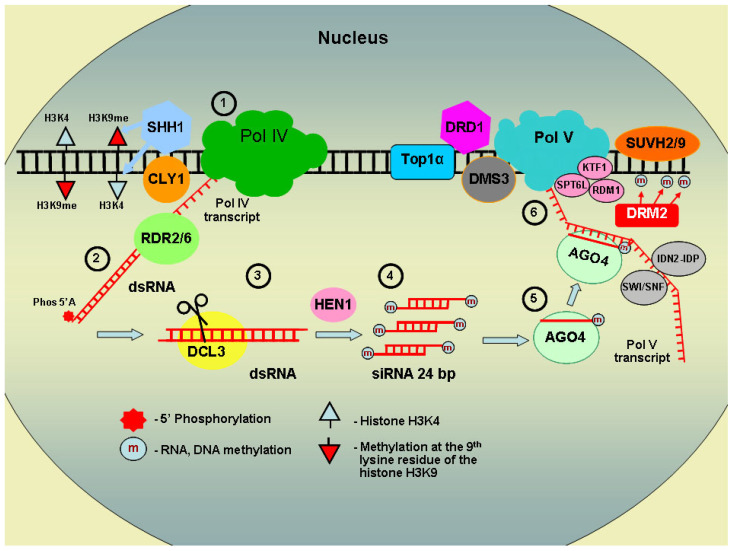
The canonical RdDM pathway. *Initiation and dsRNA Synthesis*: (1) Pol IV transcribes non-coding RNAs from target loci; its recruitment is mediated by SHH1 and the SWI2/SNF2-like factor CLSY1. SHH1 facilitates this by recognizing H3K9me and unmethylated H3K4 histone marks. (2) Pol IV transcripts are converted into dsRNA by RDR2/6. *Primary siRNA Biogenesis*: (3) dsRNA is processed by DCL3 into 24 bp siRNAs, (4) which are subsequently methylated at their 3′ ends by HEN1. *Effector Phase*: (5) These siRNAs are loaded into AGO4-containing complexes, retaining a single-stranded guide RNA. (6) Pol V, recruited by SUVH2 and SUVH9, generates scaffold transcripts that act as a docking platform for siRNA-AGO4 complexes. This assembly recruits DRM2 to catalyze DNA methylation. Access to the DNA template is ensured by TOP1α and the DDR complex (DRD1, DMS3, RDM1), while KTF1 acts as an elongation factor. The IDN2–IDP complex stabilizes the siRNA–scaffold duplex and, together with SPT6L and the SWI/SNF complex, modulates nucleosome positioning and facilitates DRM2 activity.
